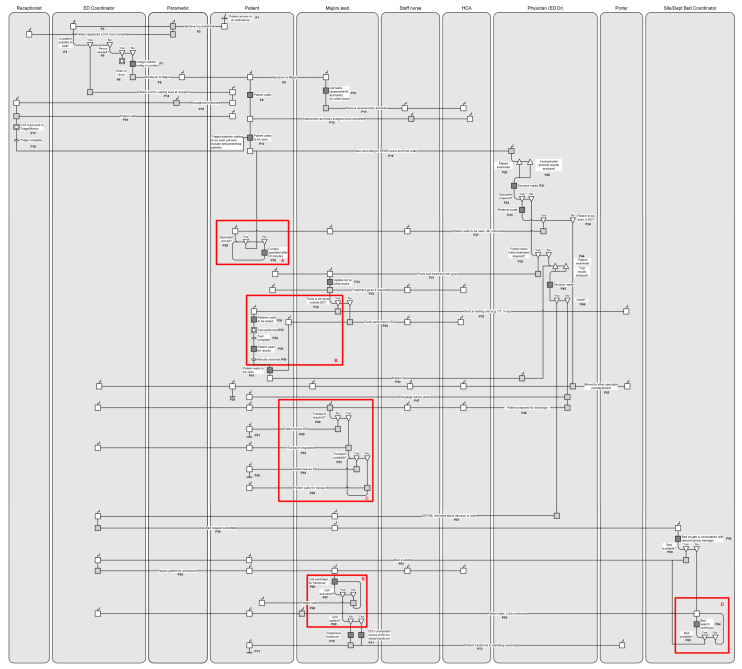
RAD of a patient’s journey through the Majors unit. Note: Phys: Physician; SEWS: Standardised Early Warning Score; CT: Computerised Tomography, CDU: Clinical Decision Unit; Resus: Resuscitation area; A: Awaiting specialist team; B: Undertaking a test outside ED; C: Awaiting transport; D: Bed search; E: Handover to admitting ward/department.
